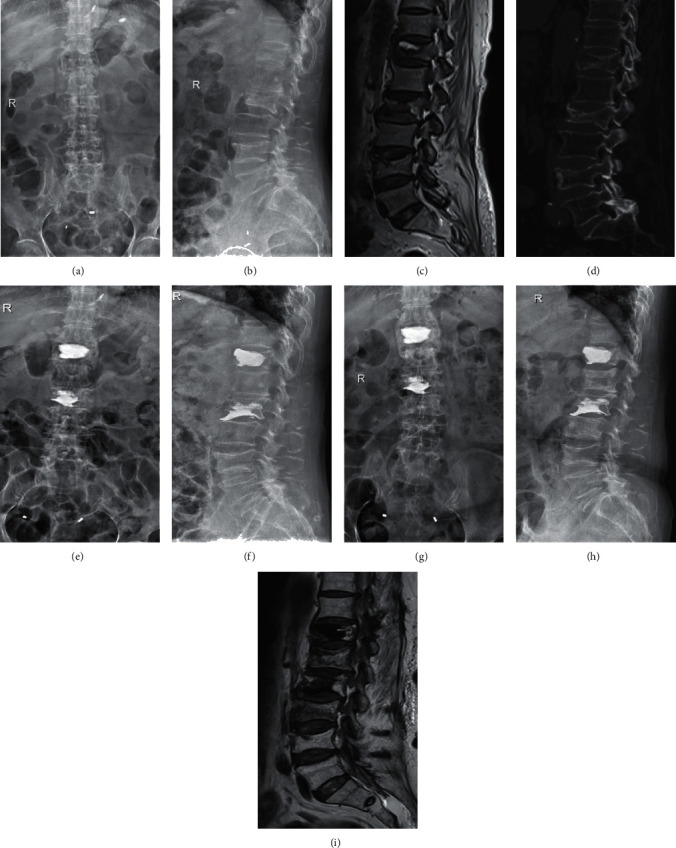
A 70-year-old woman. (a, b) Radiographs obtained in positive and lateral positions, showing a T12–L2 osteoporotic vertebral compression fracture. (c) Lumbar magnetic resonance image showing T12 and L2 vertebral body morphology changes. (d) Lumbar computed tomographic image showing T12 and L2 osteoporotic vertebral compression fractures. (e, f) Radiographs obtained in the positive and lateral positions after the first PVP operation for the T12 and L2 osteoporotic vertebral compression fractures. (g–i) At 8 months after the first treatment, the radiograph obtained in the positive and lateral positions and lumbar magnetic resonance image show new osteoporotic vertebral compression fractures at L1 and L3.
